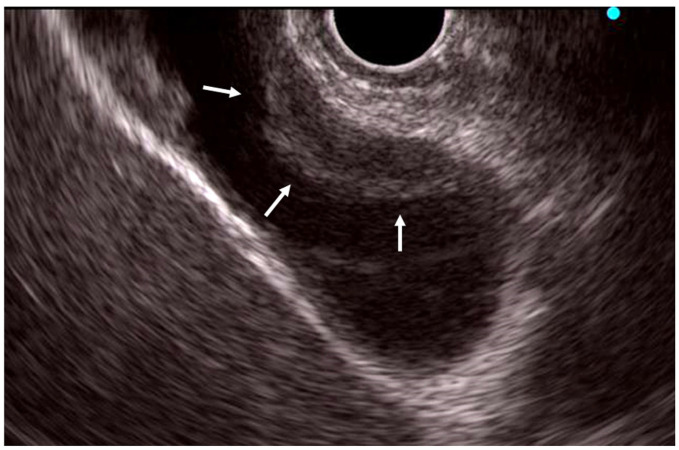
Endoscopic ultrasonographic findings showing a localized thickening of the gallbladder wall at the fundus, with the lesion located within the submucosal layer in localized IgG4-related cholecystitis (arrows).
